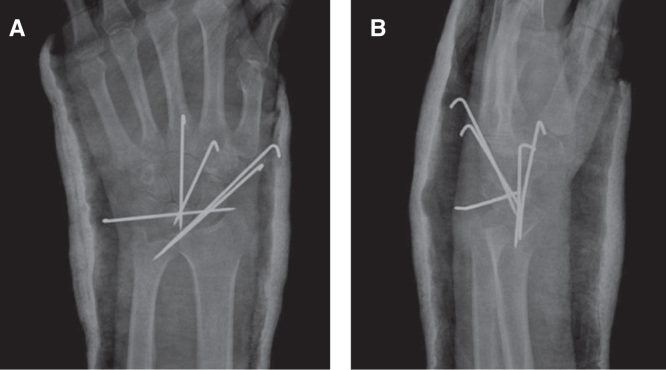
Immediate postoperative X-rays (*a* and *b*) are shown.
